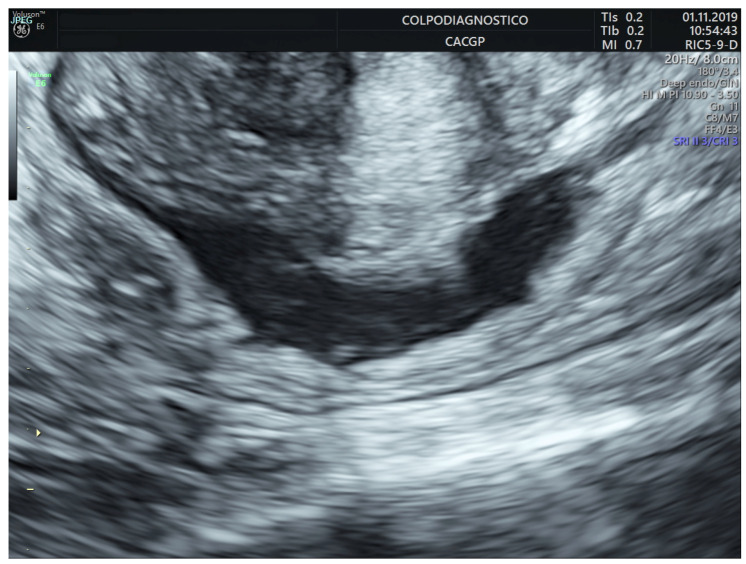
Transvaginal ultrasound image of an endometriotic nodule involving the upper rectum.
